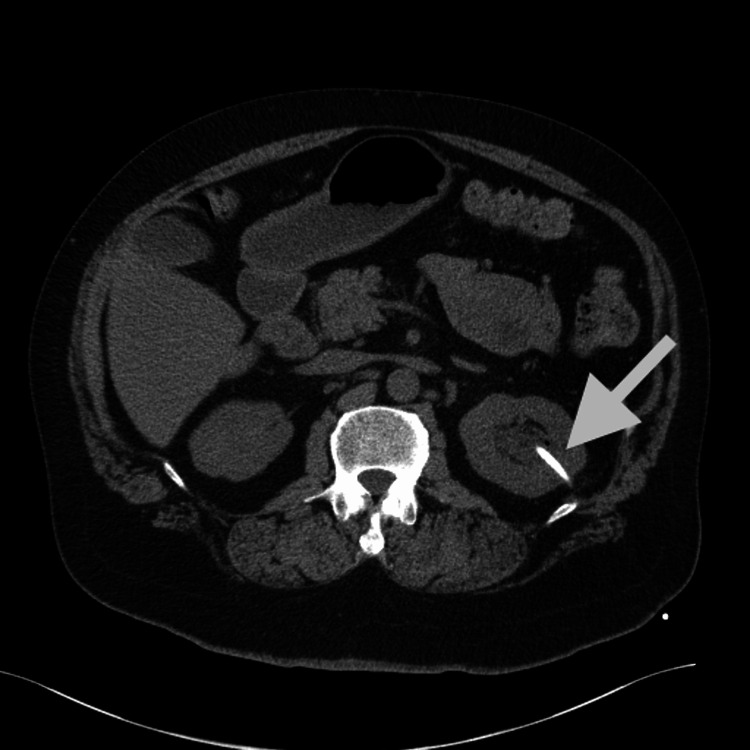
Abdominal CT scan showing the improvement of left hydronephrosis and nephrostomy tube replacing previous left ureteral stent (arrows).
